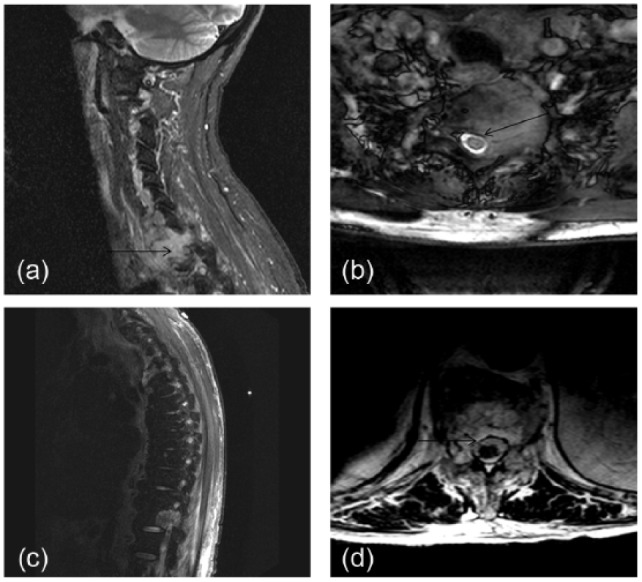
Magnetic resonance imaging of the cervical and thoracic spine. Images are taken from a study performed on the day of admission. Image “a” is a left parasagittal view of the cervical spine showing an expansile mass (arrow) in the bodies of C7 and T1 with a high T2 signal. Image “b” is a cross-sectional view of T1 showing encroachment on the vertebral canal by the lesion (arrow). Image “c” shows a similar lesion at T12. Image “d” demonstrates encroachment of this mass on the vertebral canal (arrow).
